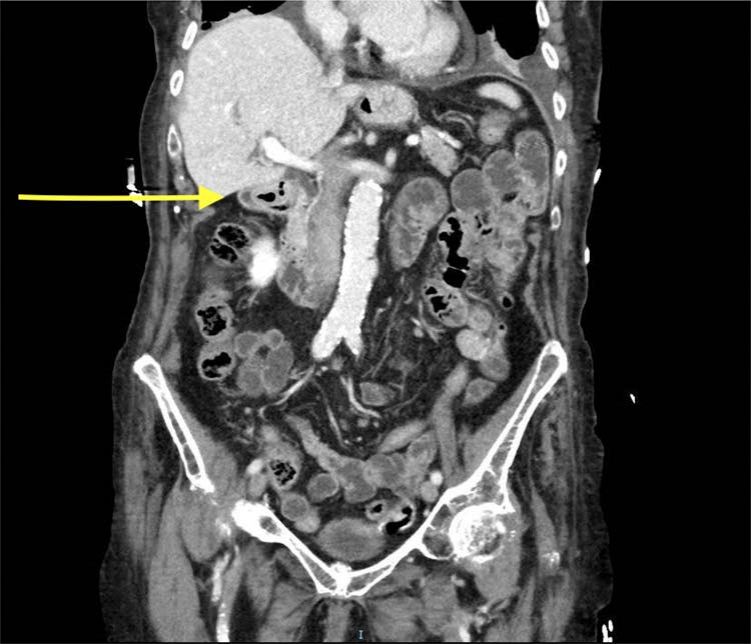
CT scan showing gastroduodenal intussusception (yellow arrow).
